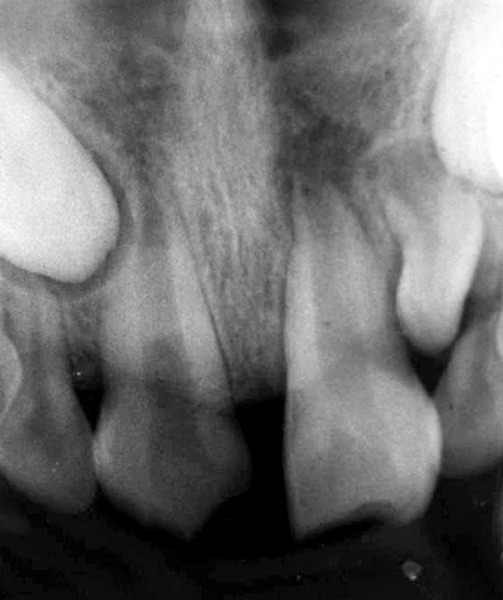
Preoperative radiograph of left central incisor with open apex and opened root canal
